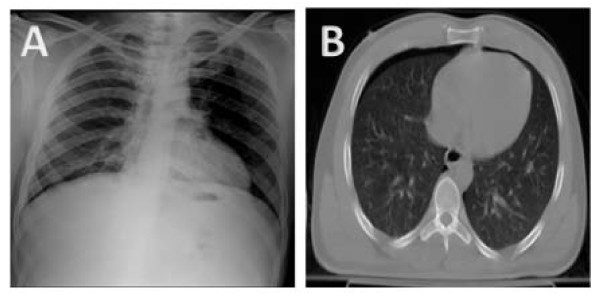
AP chest X-ray revealing no evidence of pneumothorax (Panel A). CT chest performed immediately after X-ray revealed right sided pneumothorax (Panel B).
